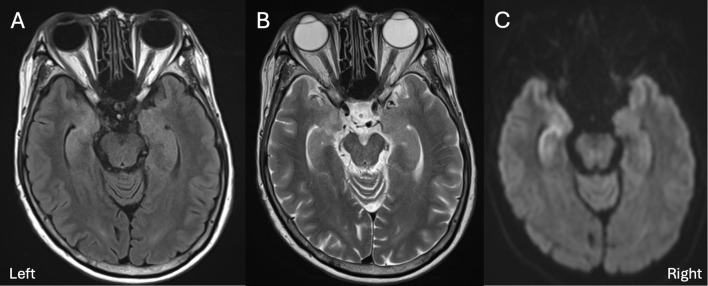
Brain magnetic resonance imaging findings at presentation. Axial fluid-attenuated inversion recovery (FLAIR) image (A) demonstrates hyperintense lesions in the bilateral medial temporal lobes, including the hippocampi and amygdalae. The corresponding T2-weighted image (B) shows similar signal abnormalities. Diffusion-weighted imaging (C) also shows hyperintense lesions in the bilateral medial temporal lobes.
